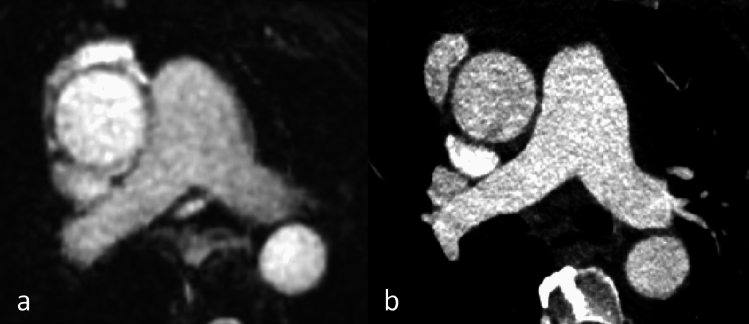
A patient presented after a near-collapse episode at rest. Hypotension and a blood pressure difference between arms were observed in the Emergency Department. The CT scan ruled out aortic dissection. Axial reformation of an enlarged view of the pulmonary arteries in MRA (a) and CTA (b). Excellent image quality in both modalities despite a lower resolution in the MRA (a)
